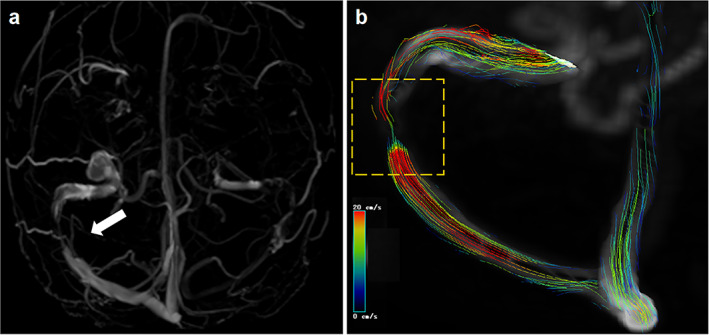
Four‐dimensional (4D) flow magnetic resonance imaging (MRI) showing the hemodynamic of cerebral venous sinus stenosis. A female presented with severe headache and papilloedema with a clinical diagnosis of idiopathic intracranial hypertension (IIH). (a) Inferior reconstruction of magnetic resonance venography (MRV) showed the drainage dominance of right transverse sinus and right transverse sinus stenosis. (b) 4D flow pathlines indicate abnormal flow condition and the existence of stenosis. The color coding of the pathlines reflects the magnitude of blood flow velocities in the vasculature (blue = low, red = high). The white arrow and yellow‐dashed square in the artwork mean the transverse sinus stenosis.
